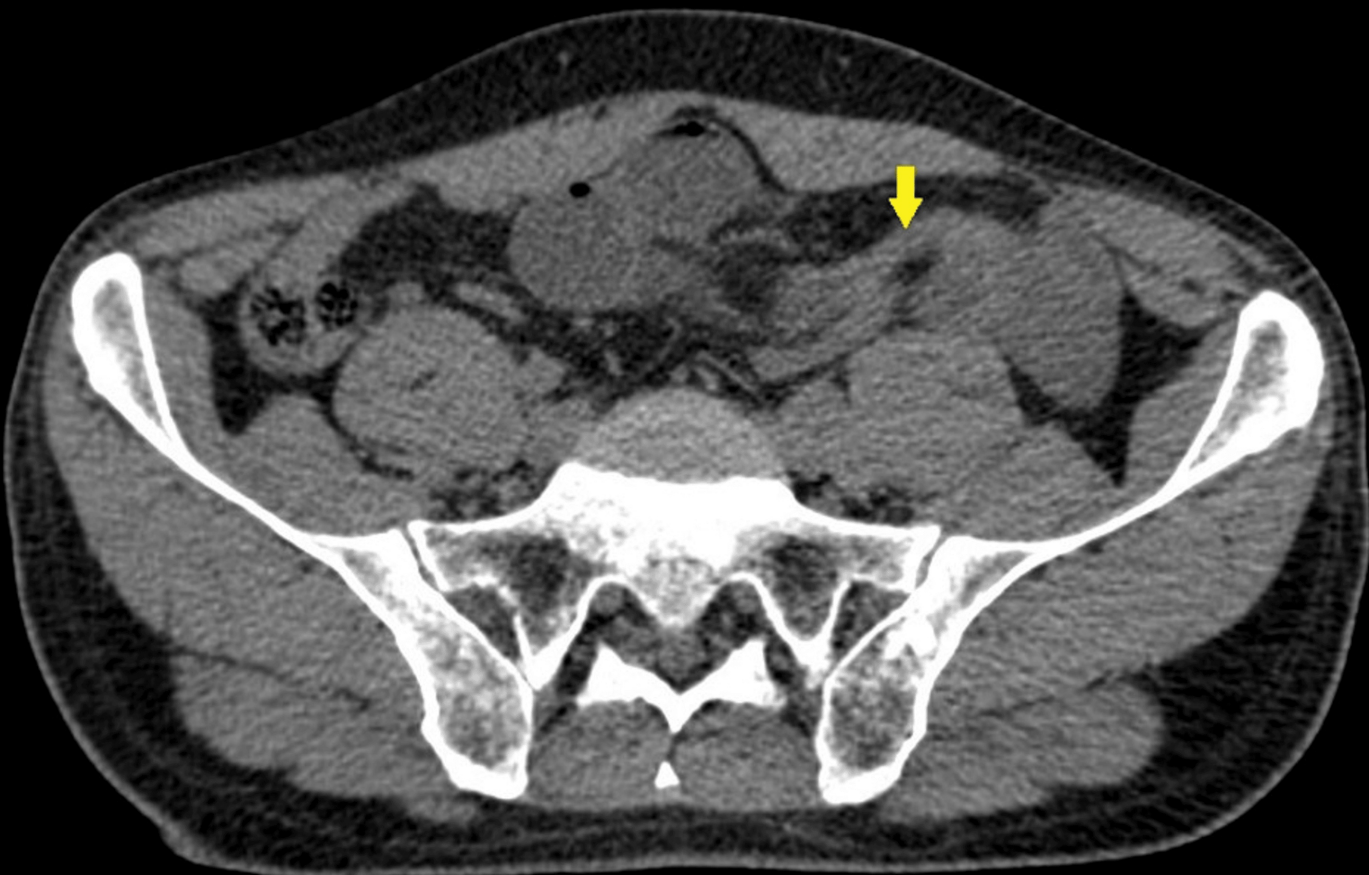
CT scan showing the transition zone in the left iliac fossa in axial section Yellow arrow: Zone of transition
